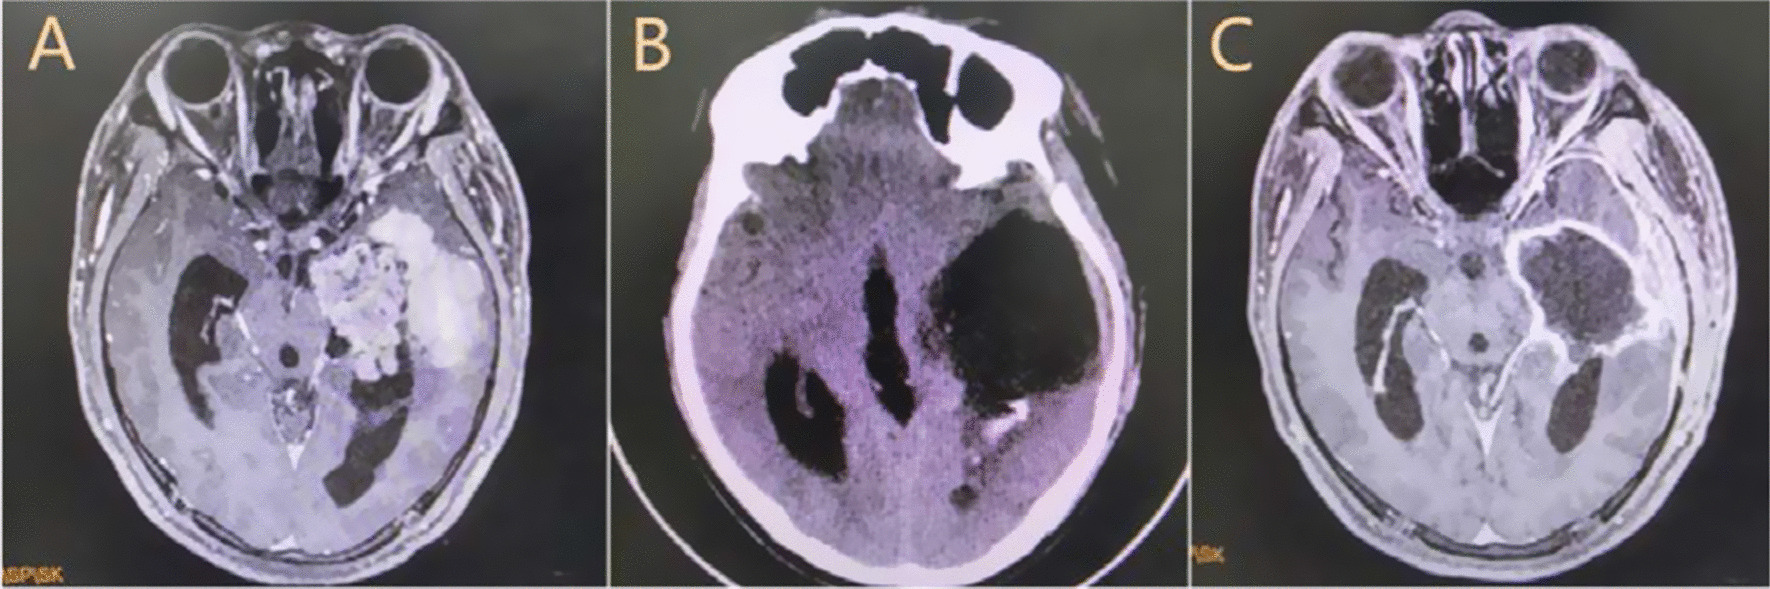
A Enhanced preoperative MRI showing a left temporal lobe-lateral ventricle tumor. B CT scans on the first day after surgery demonstrating that the tumor had been removed and replaced with CSF, with the opening of the left lateral ventricles. C Enhanced MRI images on the 43rd day after surgery demonstrating complete tumor resection
